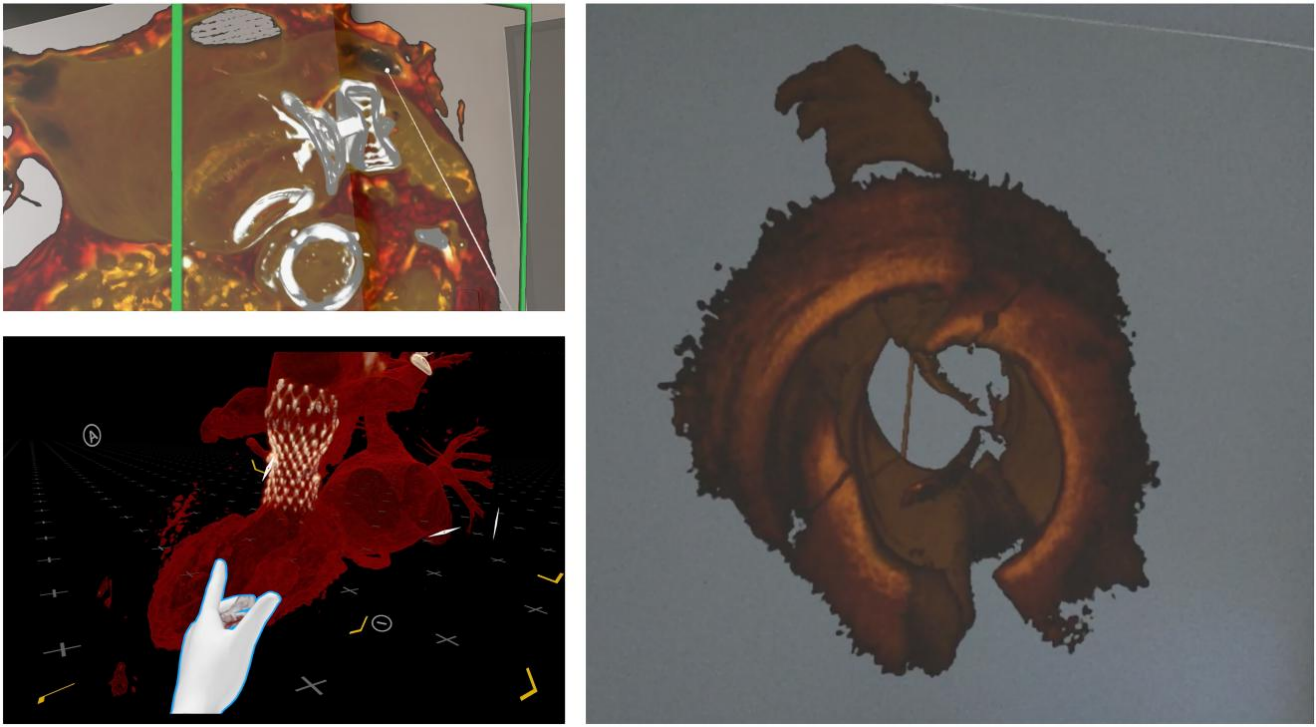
Selected example of pre-procedural and postprocedural use of Extended Realities (XR). (*A*) Evaluation of occluder implantation position post left atrial appendage closure in Mixed Reality using computed tomography images. The view presents left atrial and left atrial appendage cut longitudinally. (*B*) Result of transcatheter aortic heart valve implantation in Virtual Reality (VR). Valve frame implantation height is presented in the aortic root and left ventricular outflow tract, including the left ventricle blood pool in visualization. (*C*) Procedural planning based on Mixed Reality-based optical coherence tomography (OCT) data visualization. Vessel dissection shape and distribution are presented to the operator to plan the stenting strategy and perform spatial lumen measurements.
